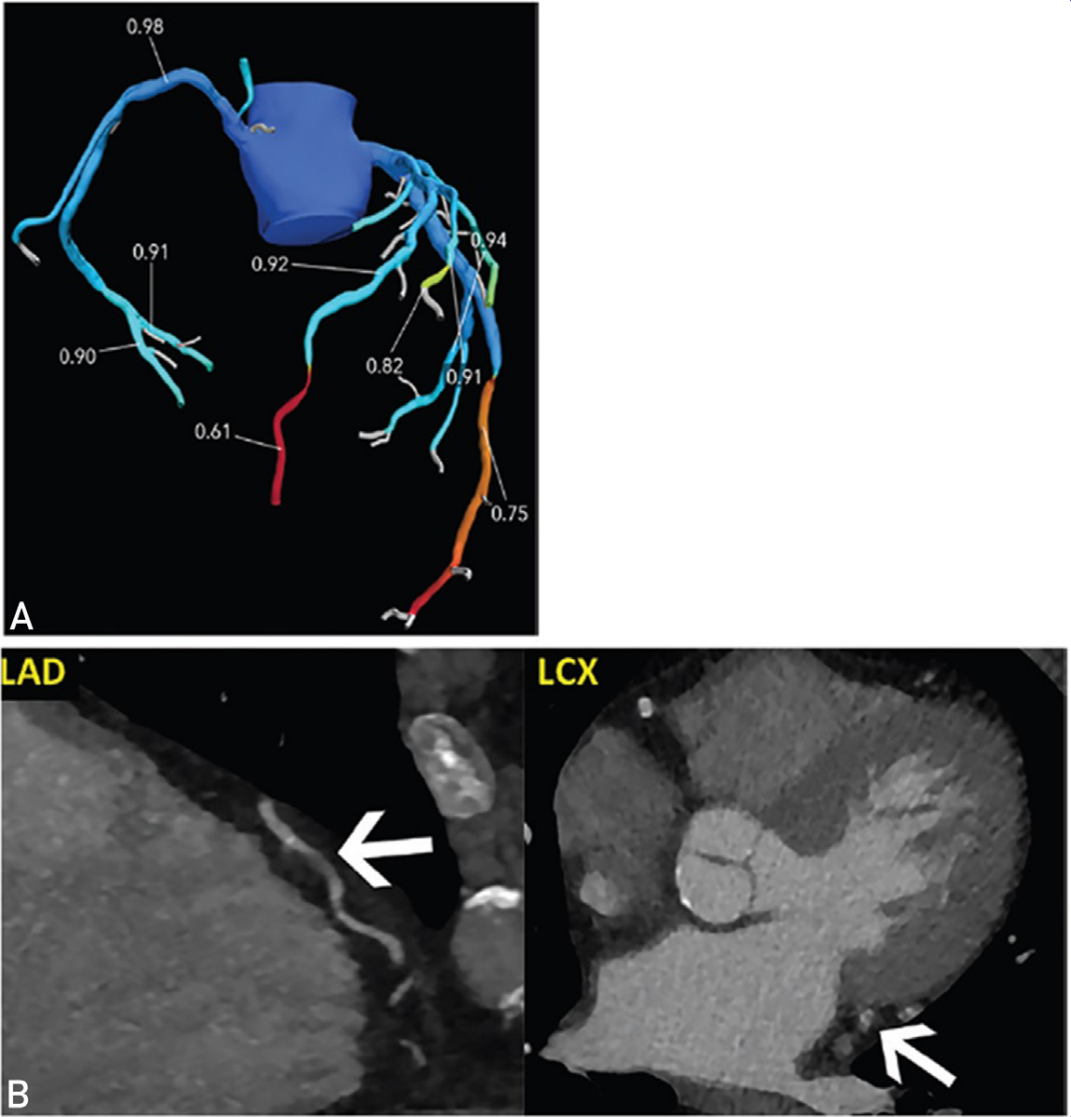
Suboptimal image quality causing artifactual CT-FFR results.^1^ A, CT-FFR image of a 73-year-old man showing positive results in LAD and LCx. B, Motion artifacts are found in LAD and LCx. These account for abnormal CT-FFR values. Pictures are adapted from Rajiah et al.^1^ CT-FFR, computer tomography–derived fractional flow reserve; LAD, left anterior descending artery; LCx, left circumflex artery.
